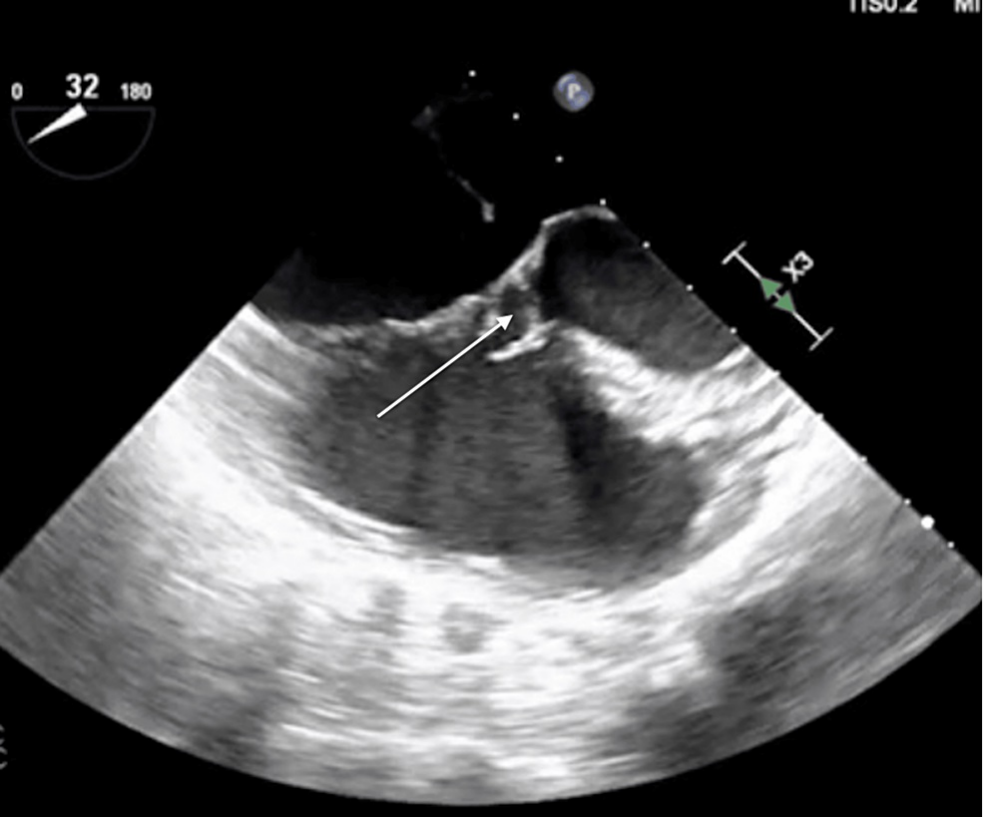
TEE mid-esophageal view showing the membranous ventricular septal defect TEE, transesophageal echocardiography
